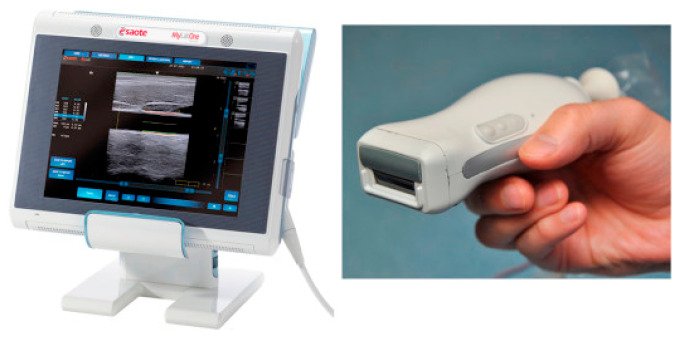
Portable imaging scanner combining photoacoustics and ultrasound. Left is the ultrasound scanner system and right is the picture of the probe integrating the laser module and ultrasound transducer array. Reprinted with permission from K. Daoudi, P.J. van den Berg, O. Rabot, A. Kohl, S. Tisserand, P. Brands, and W. Steenbergen, Optics Express, Vol.22, pp. 26365–26374, 2014; licensed under OSA’s “Copyright Transfer and Open Access Publishing Agreement” (OAPA). Copyright 2014 Optical Society of America.
